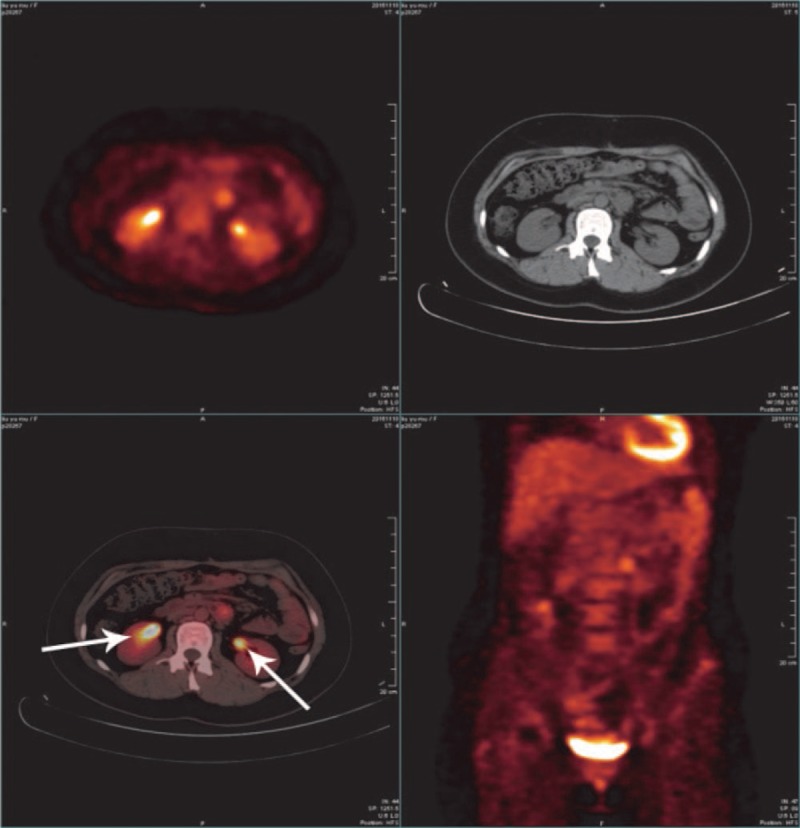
The postoperational 18F-FDG PET/CT presented 2 enlarged para-aortic lymph nodes at the level of L2 and L3 with marked accumulation of FDG and a maximum SUV of 3.5. 18F-FDG PET/CT = fluorine-18 fluorodeoxyglucose positron emission tomography/computed tomography, SUV = standardized uptake value.
